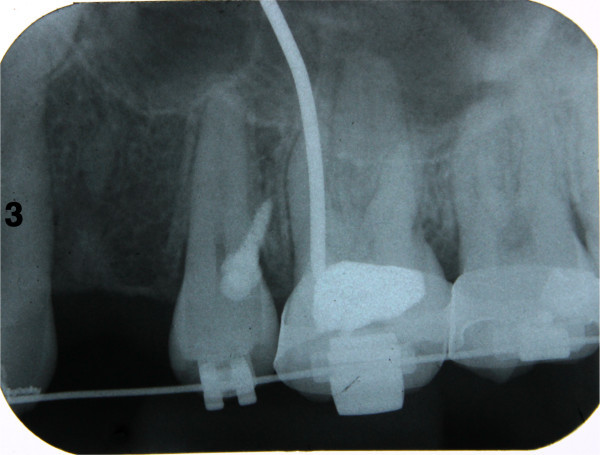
Intraoral periapical radiograph taken to check position of the mini-implant.
